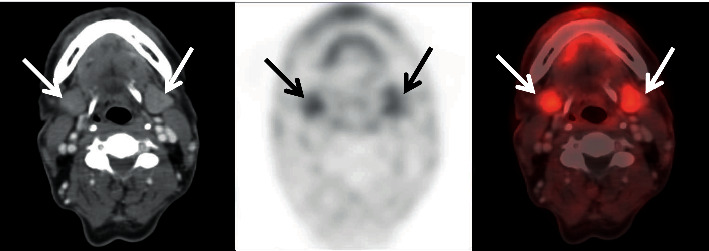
The FAPI-04 SUVmax level was 4.72 and the SUVavg level was 2.84 in the submandibular glands at axial CT, PET, and fusion images (arrows) of a 54-year-old male patient.
